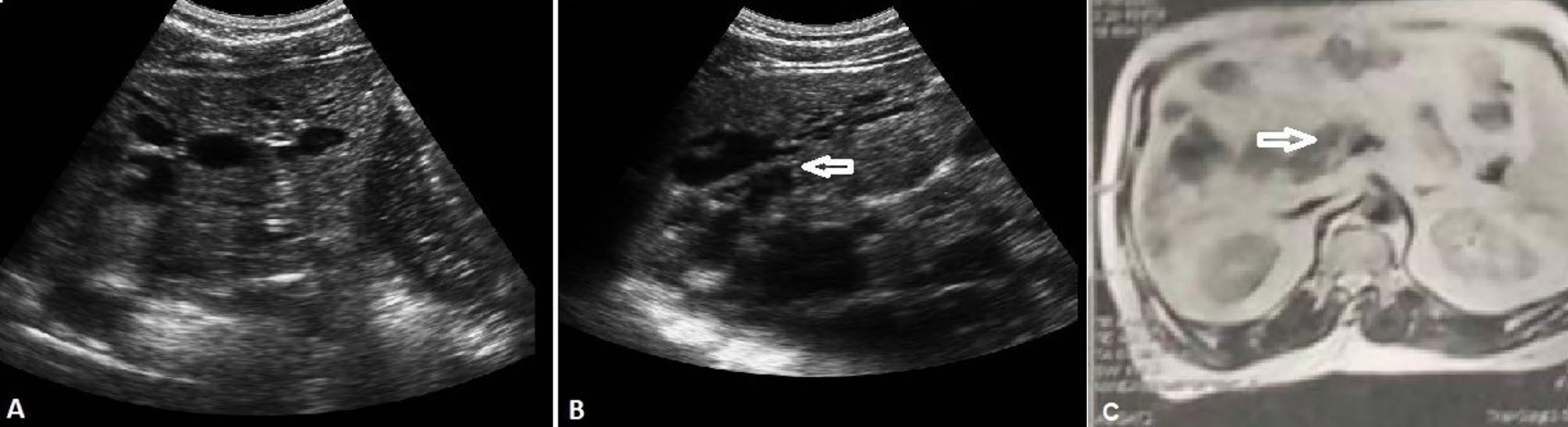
A Saccular dilation of intrahepatic ducts. B Saccular dilation of intrahepatic ducts, pancreatic cysts, and a hypoechoic mass with compressing effects (white arrow), causing dilation of intrahepatic biliary radicals. C: Triphasic CT confirming intrahepatic cholangiocarcinoma.
